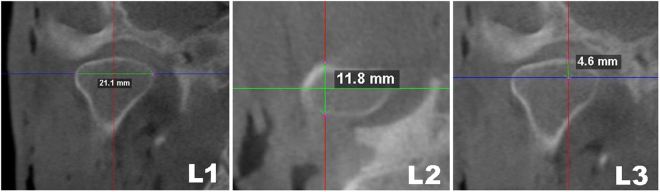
Linear measurements taken on the coronal (L1 and L3) and axial (L2) views of the CBCT image.
